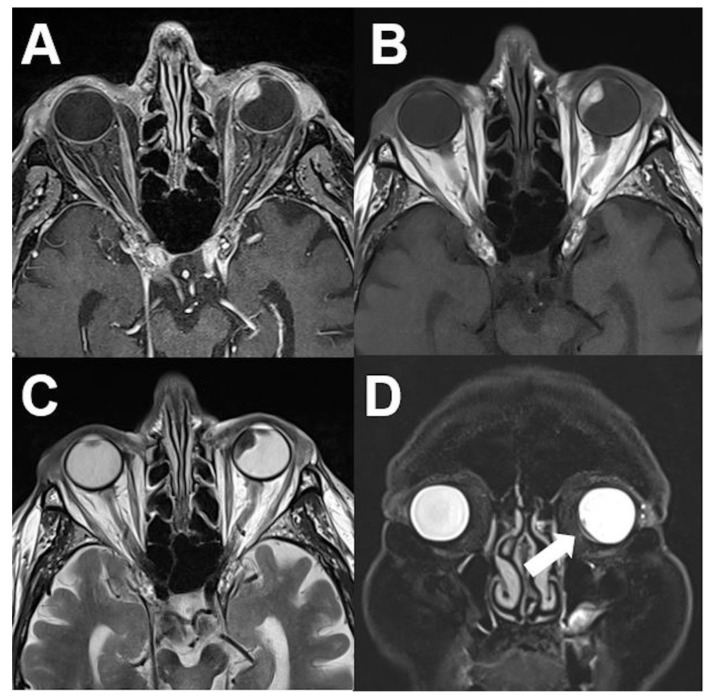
Uveal melanoma.* MRI of an 84-year-old male patient with uveal melanoma showing a well-defined tumor of the left nasal vitreous body. The tumor shows a hyperintense signal on unenhanced T1-weighted images (A), a diffuse enhancement on contrast-enhanced T1-weighted images (B), and a marked hypointense signal on T2-weighted images (C). Retinal detachment with subretinal fluid (arrows) is shown in the coronal view (fat saturated T2-weighted sequence) (D).
